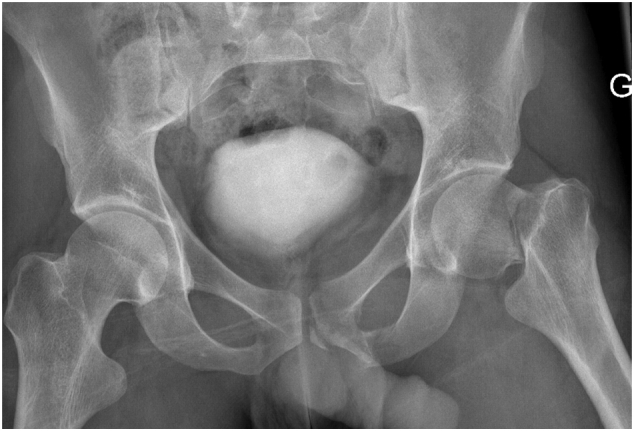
AP pelvis radiograph shows a left Pauwels type II femoral neck fracture.
